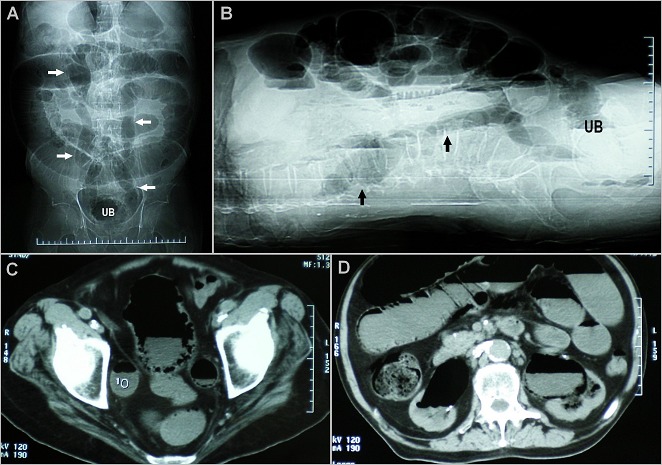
(A) supine frontal; (B) supine cross-table lateral abdominal radiographs showing linear air distribution along the collecting system of both kidneys, the ureters and around the urinary bladder (UB); (C) computerized tomography scan pelvis-sagittal section showing intramural air with intraluminal air-fluid level in the urinary bladder and the ureters; (D) computerized tomography scan abdomen-sagittal section showing intramural air with intraluminal air-fluid level in the pelvicalyceal systems. There were no gas pockets or fluid collections seen within the renal parenchyma or in the perinephric tissues
